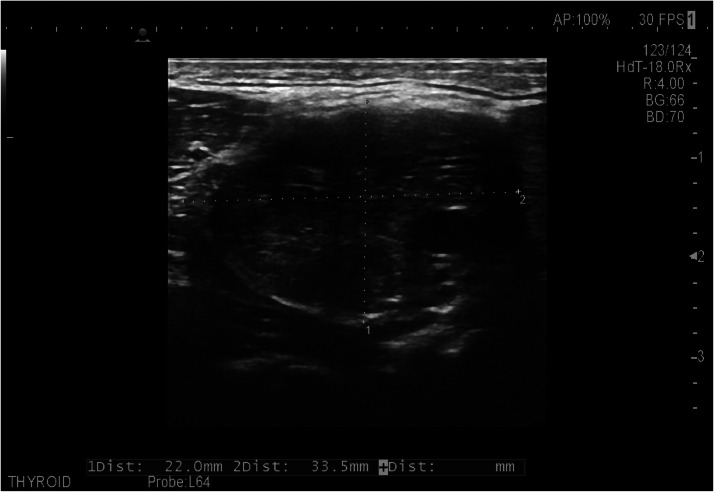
Grey scale ultrasound image showing well defined isoechoic mass in left carotid triangle measuring 33.5 × 22 mm.
